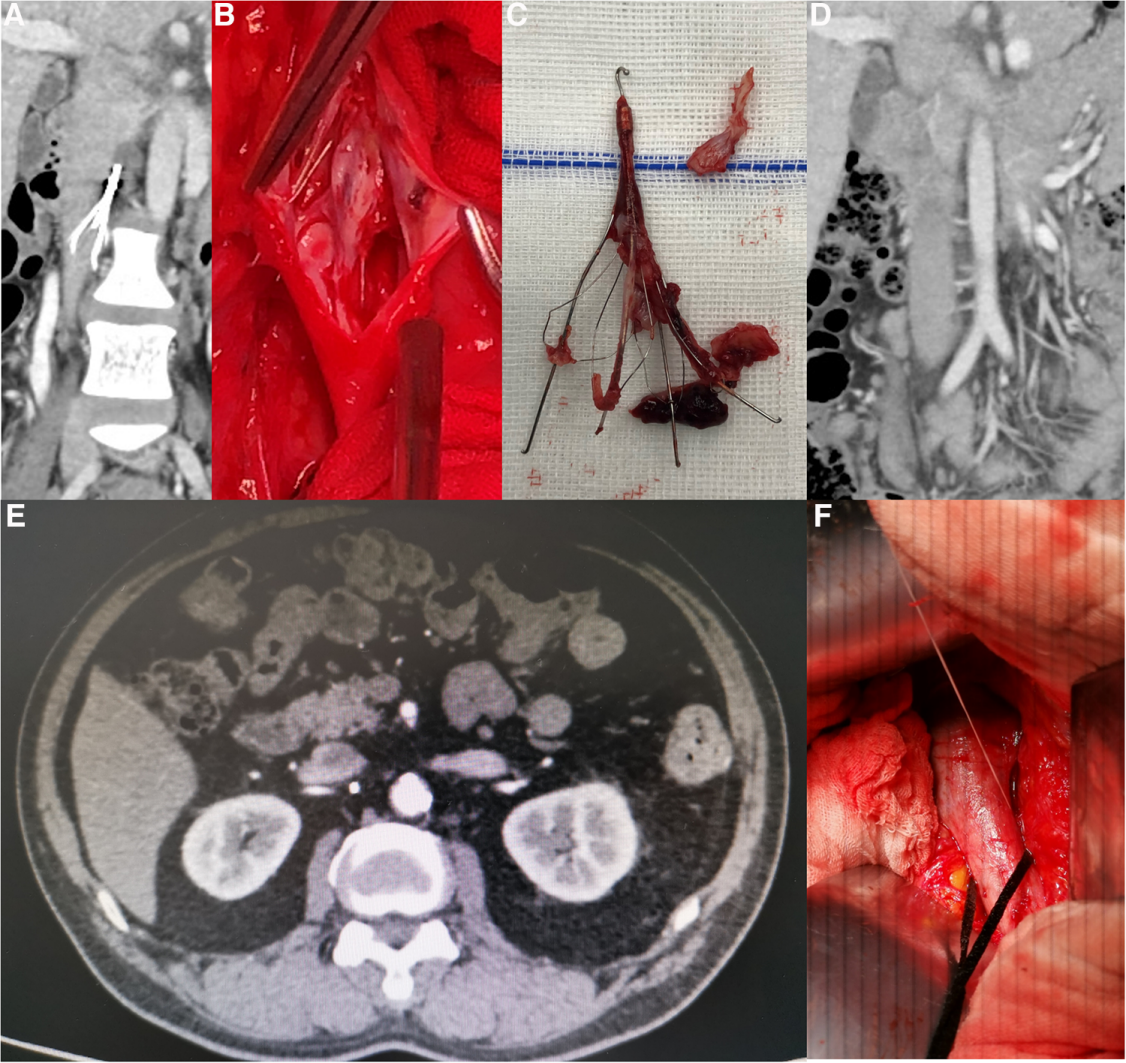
(A) Retention of Tulip filter for 16 months. (B) Tulip filter with obvious endothelial hyperplasia and failed endovenous removal. (C) Complete removal of Tulip filter with severe endothelial hyperplasia. (D) Follow-up of Tulip filter removal at 6 months after surgery with patent IVC. (E) CT image of the Celect filter showing the retrievable hook penetrating the IVC. (F) Intraoperative retrievable hook penetrating the venous wall with a preplaced purse-string suture.
